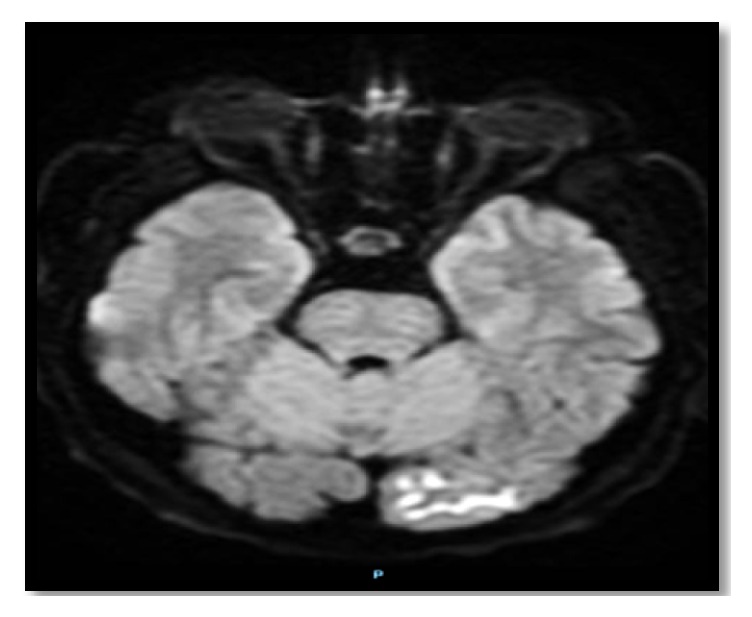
Periictal brain MRI DWI showing gyriform hyperintense signal over the left occipital cortex. Abbreviations: DWI, diffusion-weighted image; MRI, magnetic resonance imaging.
